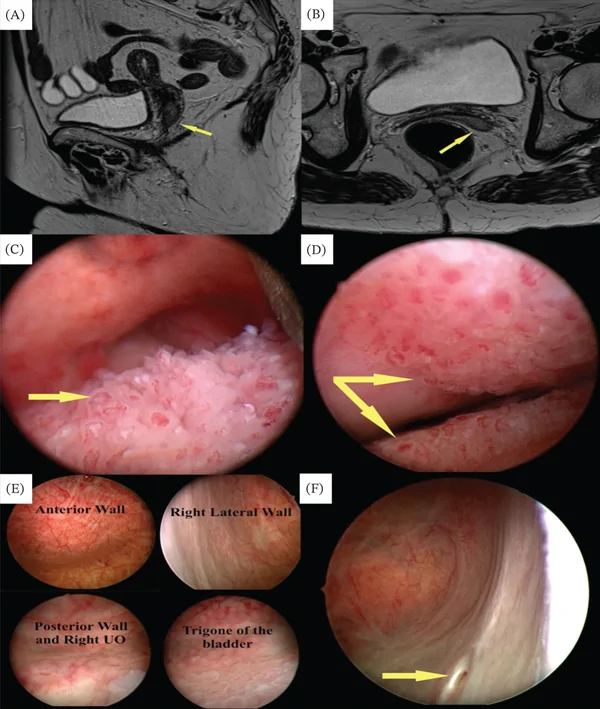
MRI scan showed asymmetrical thickening of the left side of the vagina (yellow arrows) consistent with a vaginal lesion and abnormal tissue in the fat plane between the anterior vagina and the posterior bladder wall suspicious for paravaginal tissue involvement (A: sagittal view, B: transverse view). (C, D) Colposcopy showed a papillary tumour (yellow arrows). (E) Cystoscopy showed a normal appearance of anterior wall, right lateral wall, posterior wall, right ureteric orifice and trigone. (F) The left lateral wall of the bladder demonstrated scarring from the previous TURBT with the left ureteric orifice visible in the region of scarring (yellow arrow).
